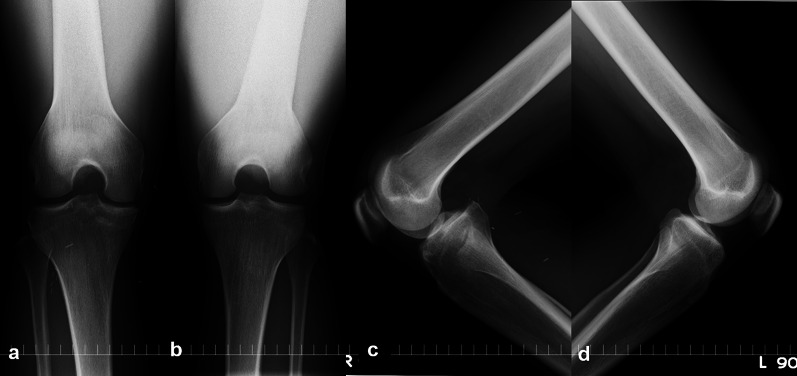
Postoperative radiograph (same patient as in Fig. 2). a, b Radiograph taken 4 years after primary repair showing no significant change in osteoarthritis; c, d posterior sag view of bilateral knee. Radiograph of right knee shows posterior laxity of 13 mm. This patient does not need further surgery and has returned to recreational baseball play without restriction
